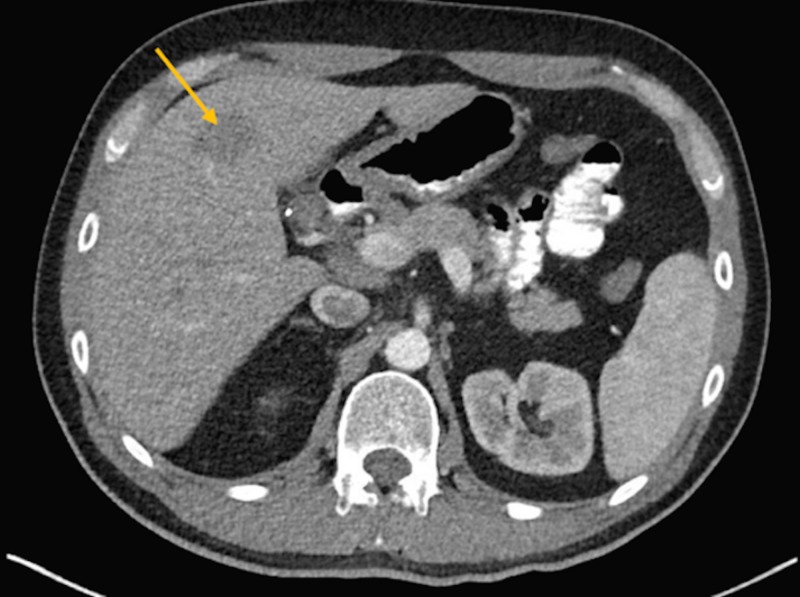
Computed tomography (CT) scan of the liver showing a mass in the left lobe
